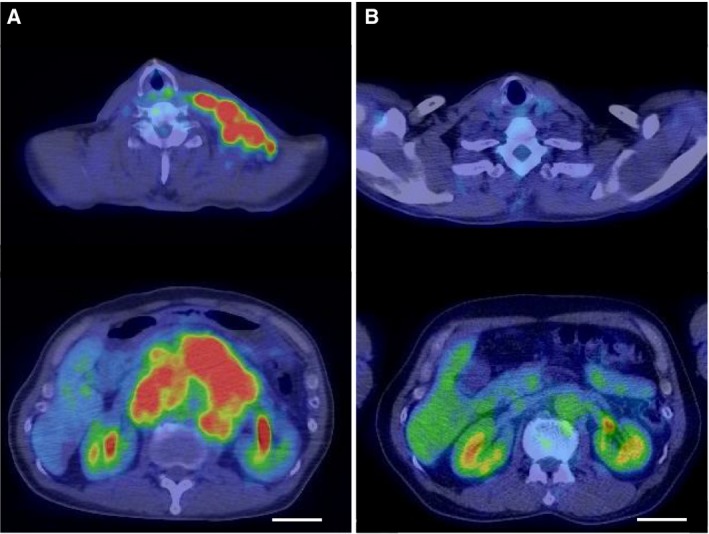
Radiological examination of FDG‐PET. (A) FDG‐PET showed abnormal uptake in regions with cervical and celiac multiple lymphadenopathy; (B) After 14 cycles of S‐1 plus cisplatin therapy, FDG‐PET showed no abnormal uptake (scale bar 5 cm).
